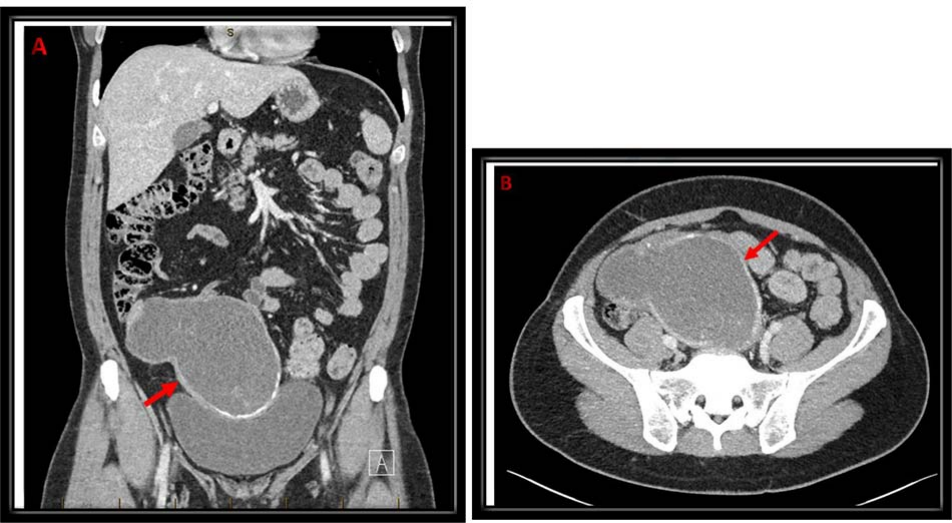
Coronal (A) and transverse (B) cuts of CT abdomen/pelvis demonstrating a large complex mass (red arrow) extending from the central pelvis to the right lower quadrant of the abdomen with some calcifications at the inferior and medial edges with some internal calcifications.
